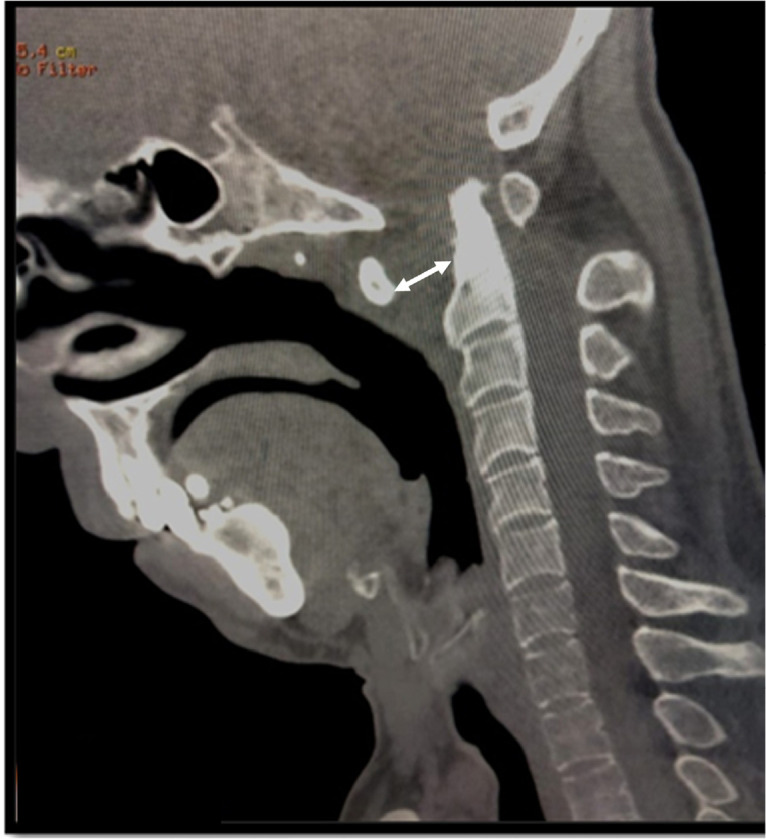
Sagittal section of cervical spine CT scan showing a C1-C2 diastasis of 15mm, suggesting an anteroposterior subluxation with a rupture of the transverse ligament.
